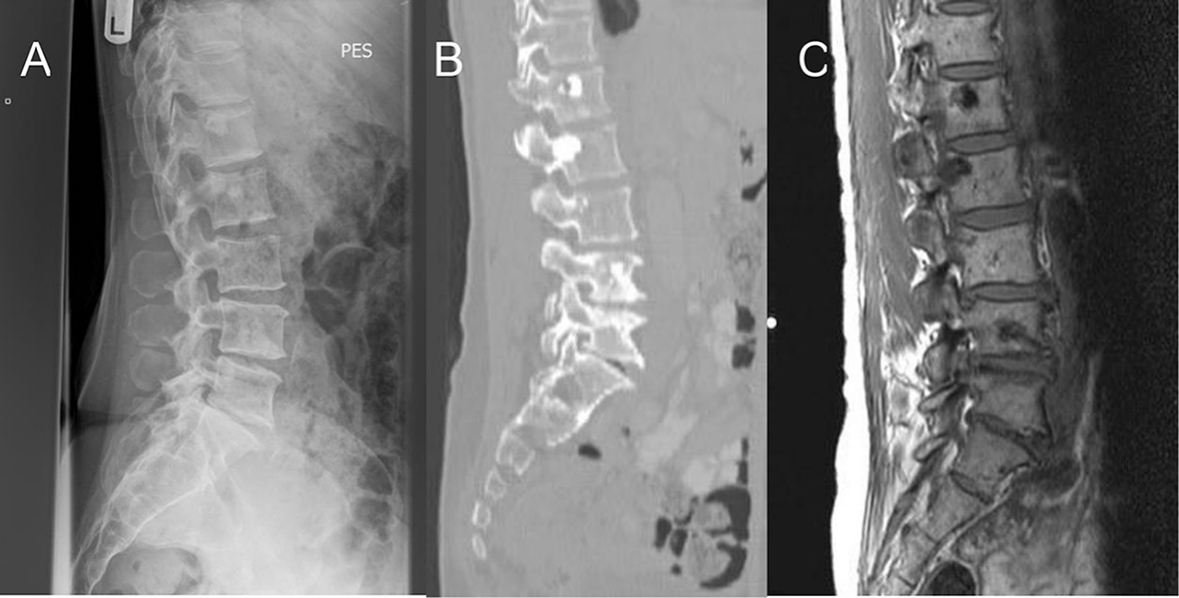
Additional imaging requested to investigate increased density in the lumbar spine on DEXA scan of a 57-year-old female with early-stage breast cancer (Case 1). (a) X-ray of lumbosacral spine showing focal bony sclerosis at T12 and L1. (b) Sagittal section of CT of lumbosacral spine showing sclerotic lesions in L1 to L4. (c) Sagittal *T*_1_ section of MRI of lumbosacral spine showing corresponding low signal lesions in the vertebral bodies.
